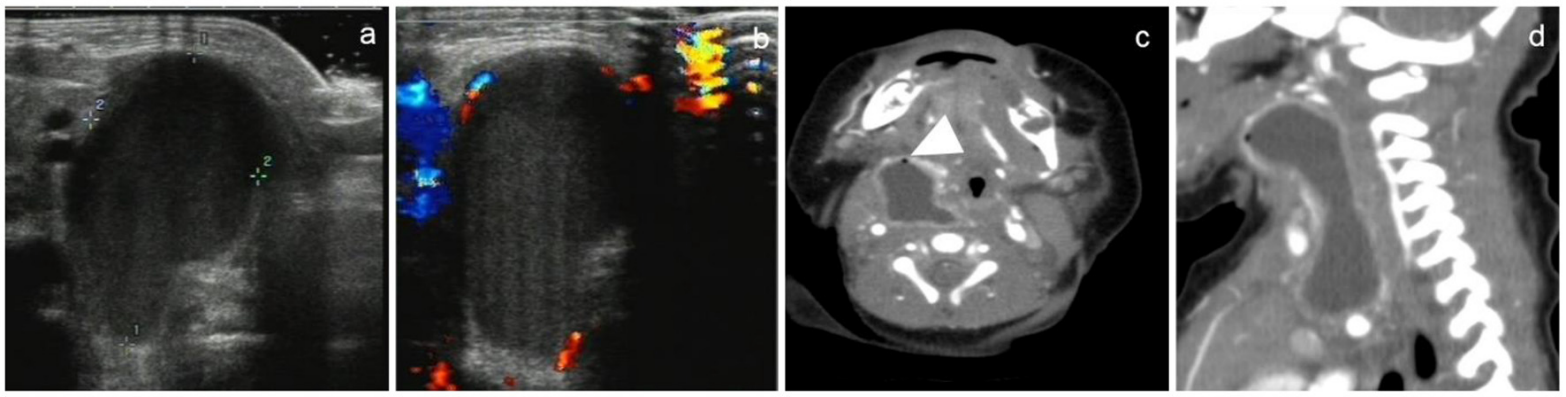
A 15-day-old female newborn with CPSF on the right neck. US and CT showed a non-septal cystic mass in the right neck. US showed an inhomogeneous mass with low echogenicity, in which there were no air bubbles (a). Color Doppler showed there was no blood flow sonogram in the cyst (b). CT showed that the cyst wall was thick and high attenuation, the border of the cyst was poorly defined, and tiny air bubbles could be seen in the cyst (arrowhead). The cystic mass extended laterally into the retropharyngeal space (c) and longitudinally into the anterior mediastinum (d).
